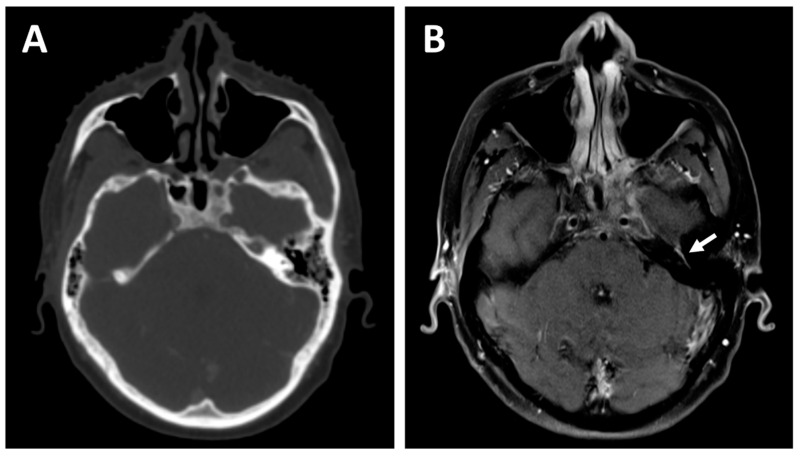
A 27-year-old patient with a cT4N2 undifferentiated carcinoma of the nasopharynx who received 3 cycles of Cisplatin and Gemcitabine induction chemotherapy before concurrent chemoradiotherapy. The planning contrast-enhanced computed tomography (CT) (A) demonstrates excellent response to the induction treatment but fails to detect residual perineural involvement of the left facial nerve, visualized as an asymmetrical enhancement on the planning T1-weighted contrast-enhanced magnetic resonance imaging (MRI) (B)—white arrow. The facial nerve involvement could be tracked down to the left parotid and was consequently included in the radiation treatment volume.
